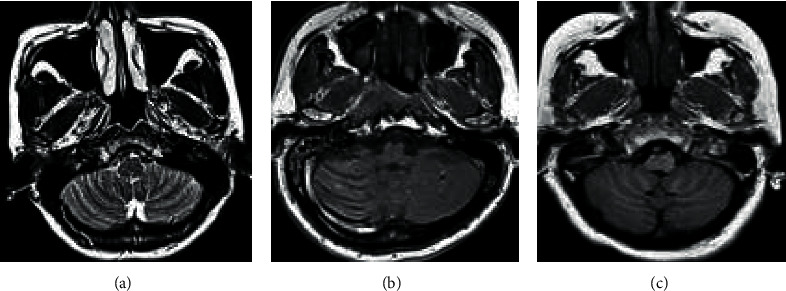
MRI imaging manifestations of some patients and healthy people. (a) An MRI image of a male patient with NC (aged 28 years old); (b) an MRI image of a female patient with NC (aged 35 years old); and (c) a normal nasopharyngeal MRI image of an adult (aged 25 years old).
